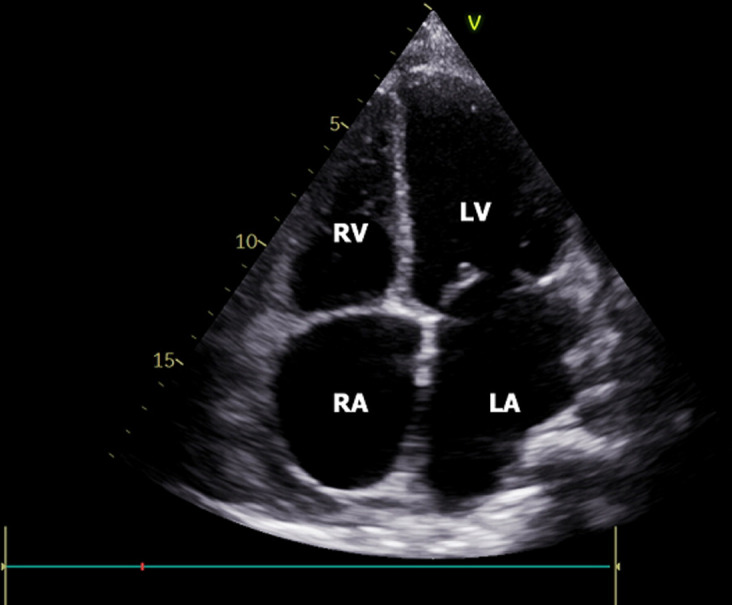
the follow-up echocardiography after pericardiocentesis
